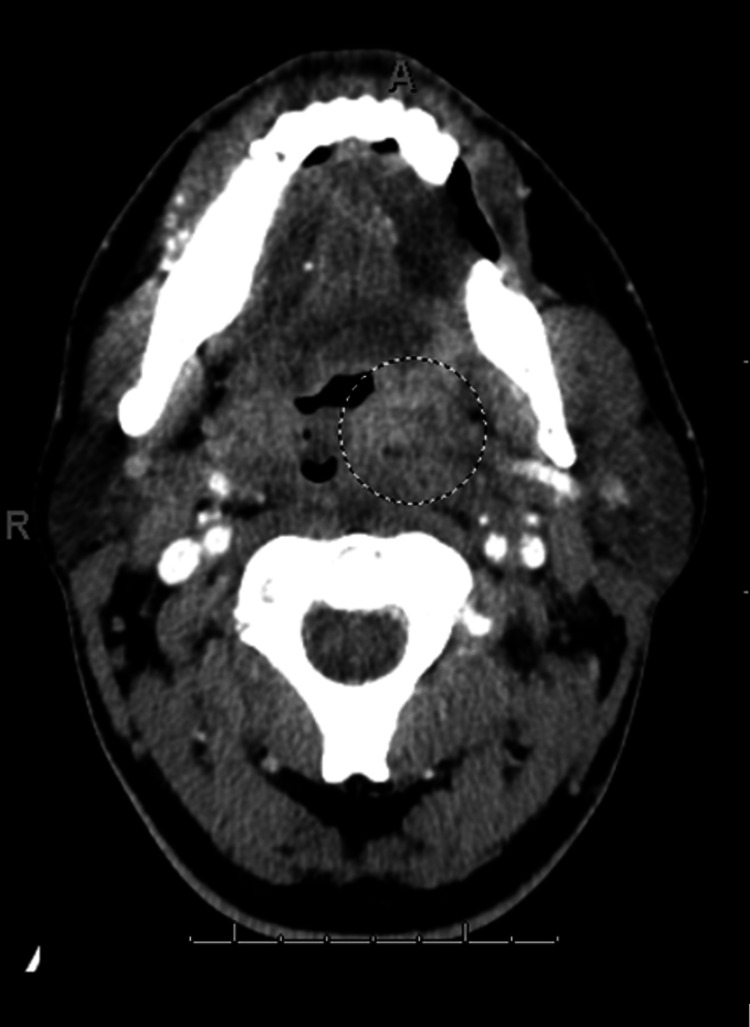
Initial axial contrast-enhanced CT image of the neck showing soft tissue swelling and edema in the oropharyngeal space, indicated by the circle. There is also enlargement of the left palatine tonsil.
